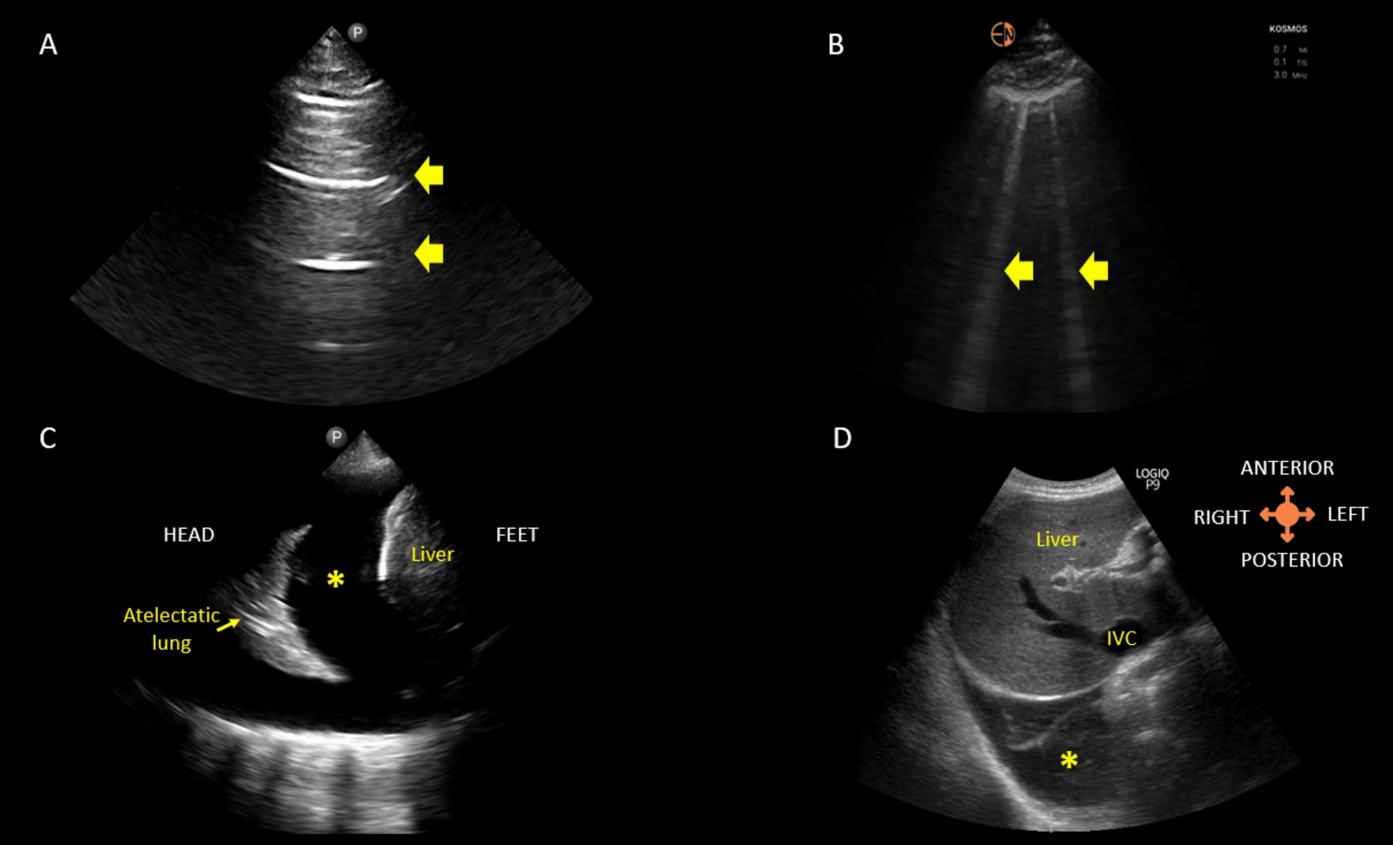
LUS (A) Normal lung showing horizontal artifacts, i.e., A-lines (arrows). (B) Vertical artifacts (arrows) known as B-lines indicate interlobular septal thickening, typically seen in congestion. (C) Pleural effusion (asterisk) as seen on a lateral scan. (D) Right pleural effusion (asterisk) as seen from the subxiphoid scanning window. IVC: inferior vena cava; LUS: lung ultrasound Turk et al. (2023) [66]; Creative Commons Attribution (CC BY) license
